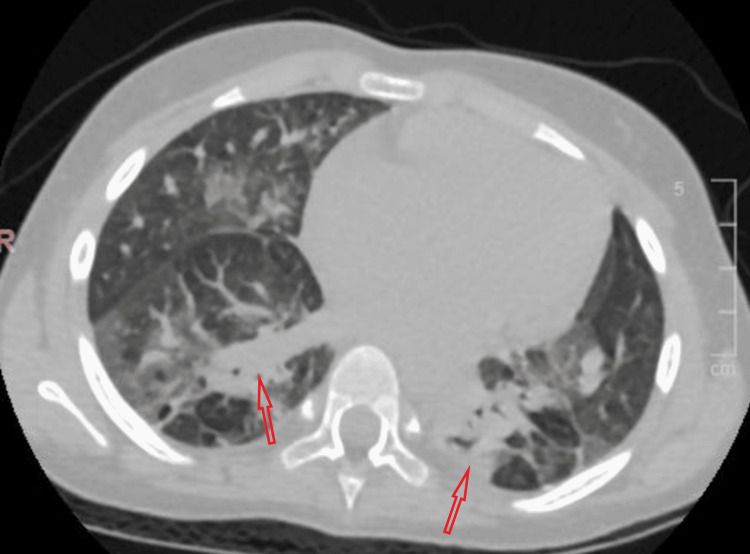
CT chest showed bilateral consolidation (red arrows) with air bronchogram.
